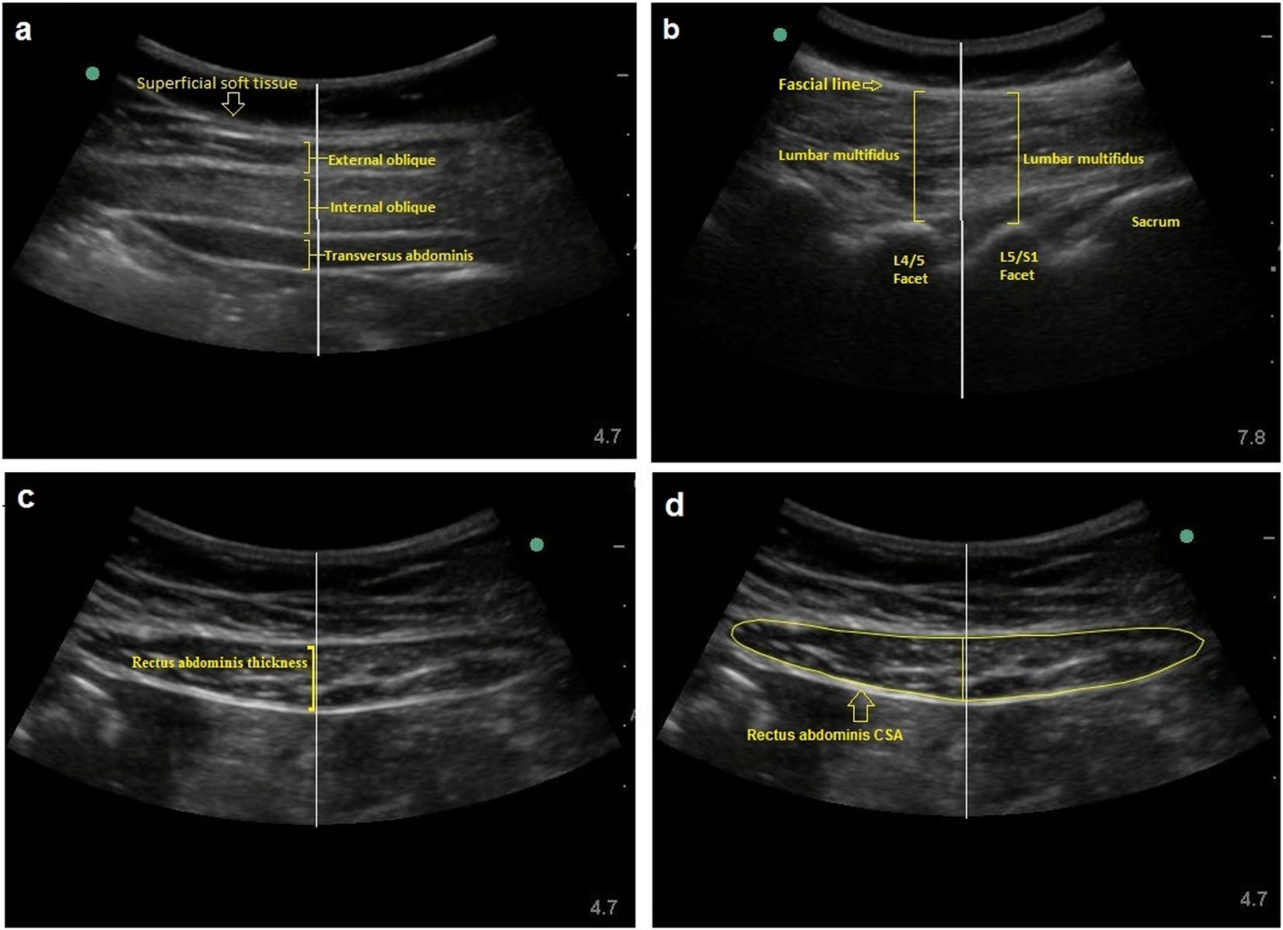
Ultrasound images of the lateral, anterior abdominal and posterior trunk muscles at rest. (a) Thickness measurements of lateral abdominal muscles were made between the superficial and deep borders of the external oblique, internal oblique, and transversus abdominis muscles in the middle of the muscle belly; (b) Thickness measurements of lumbar multifidus were made between the posterior-most portion of the L4/L5 and L5/S1 facet joints, and the plane between the muscle and subcutaneous tissue; (c) Measurement of the rectus abdominis muscle thickness was obtained between the deep and superficial borders of the rectus abdominis muscle; (d) Measurement of the cross-sectional area of the rectus abdominis muscle was obtained by tracing the interior border of the rectus abdominis muscle.
